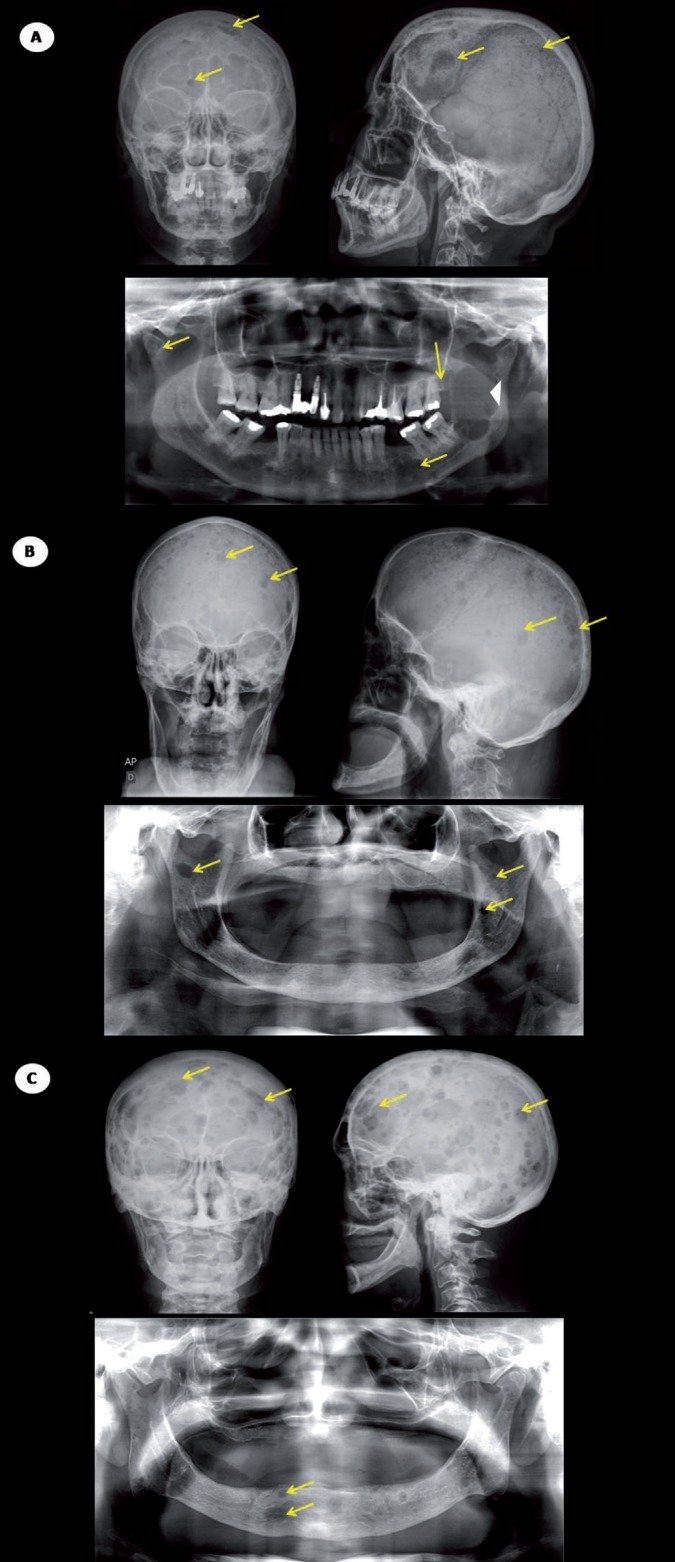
A. Digital panoramic radiography showing punched-out lesions (arrows) affecting maxilla and mandible and a large osteolytic image (arrowhead) affecting the left ramus of the mandible. Frontal and lateral skull radiographs of the same patient presenting multiple punched-out lesions (arrow). B. Digital panoramic radiographic evaluation showing punched-out lesions (arrows) affecting maxilla and mandible. Frontal and lateral skull radiographs of the same patient presenting multiple punched-out lesions (arrow). C. Digital panoramic radiographic evaluation showing punched-out lesions (arrows) affecting maxilla and mandible. Frontal and lateral skull radiographs of the same patient presenting multiple punched-out lesions (arrow).
